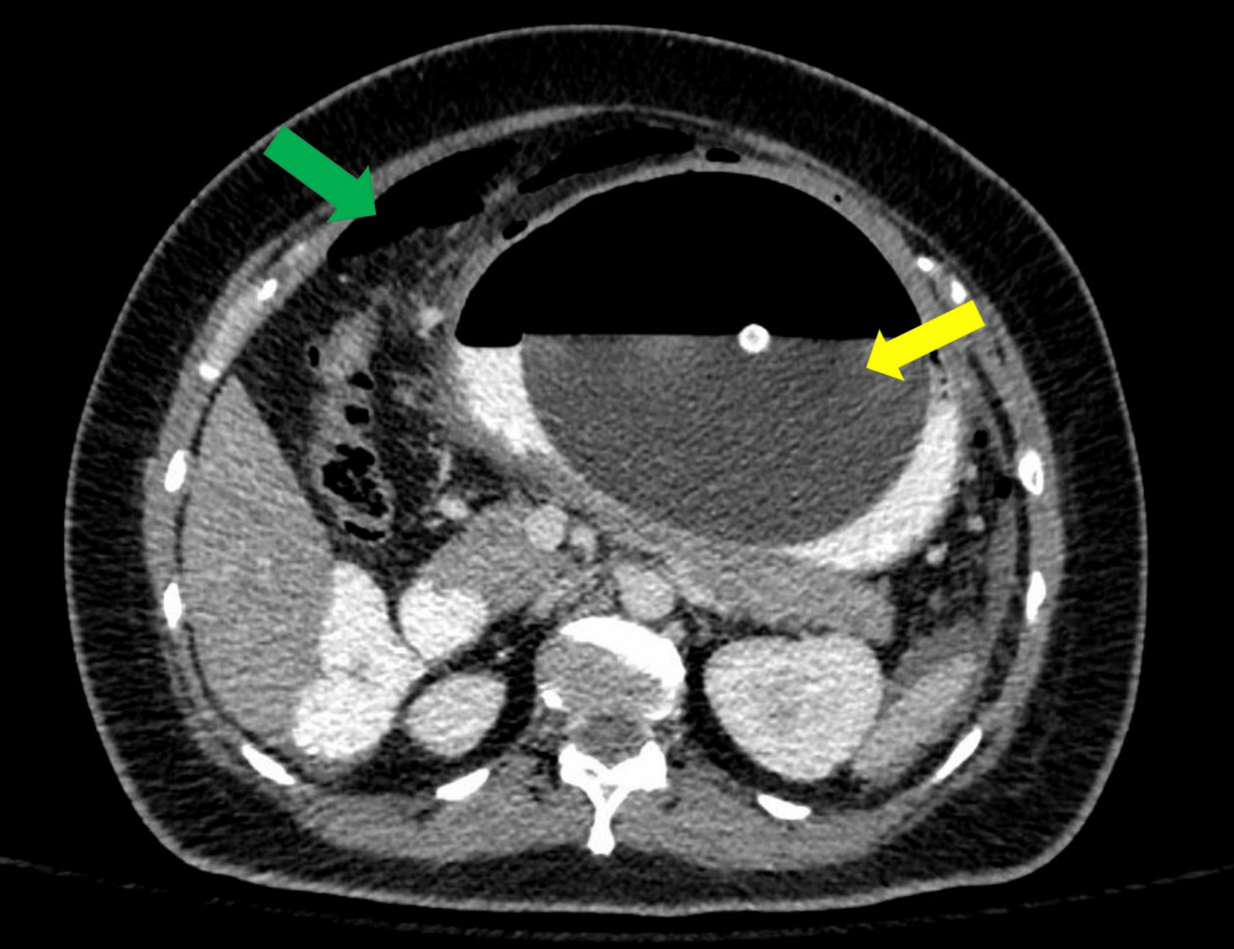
Contrast-enhanced CT of the abdomen (axial view) showing the hyperinflated intragastric balloon within the stomach (yellow arrow) with surrounding oral contrast and pneumoperitoneum (green arrow).
